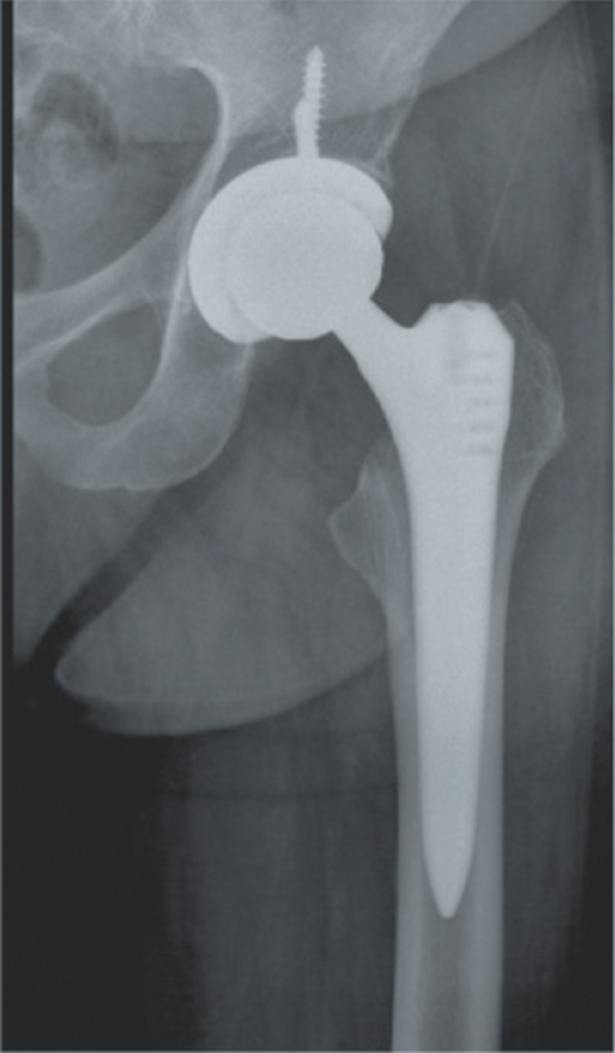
X-Ray view of the total hip arthroplasty (THA).
